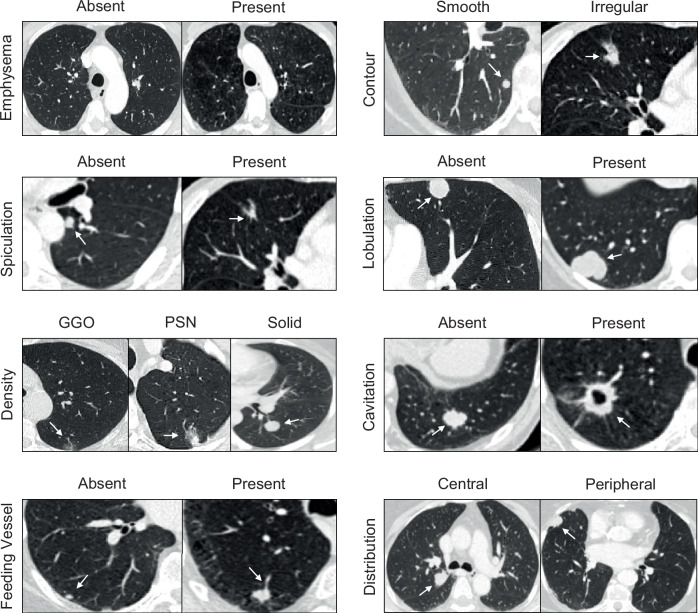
Radiologist-defined semantic features assessed on CT imaging from top to bottom row with arrow highlighting ROI: emphysema (absent/present), contour type (smooth/irregular), spiculation (absent/present), lobulation (absent/present), density (GGO/PSN/solid), cavitation (absent/present), feeding vessel (absent/present), distribution* (central/peripheral). GGO, ground glass opacity; PSN, part-solid nodule; ROI, region of interest
